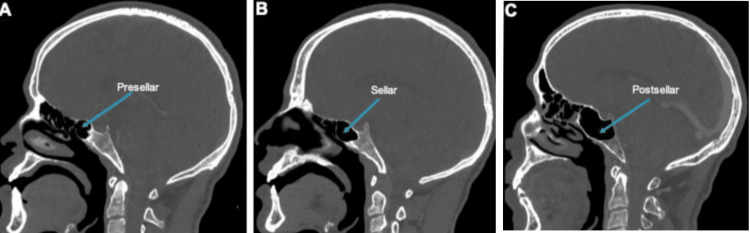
Sagittal section of a CT scan showing pneumatization of the SS. (A) Presellar pattern, (B) sellar pattern, and (C) conchal pattern of pneumatization (blue arrows) CT: computed tomography; SS: sphenoid sinus
